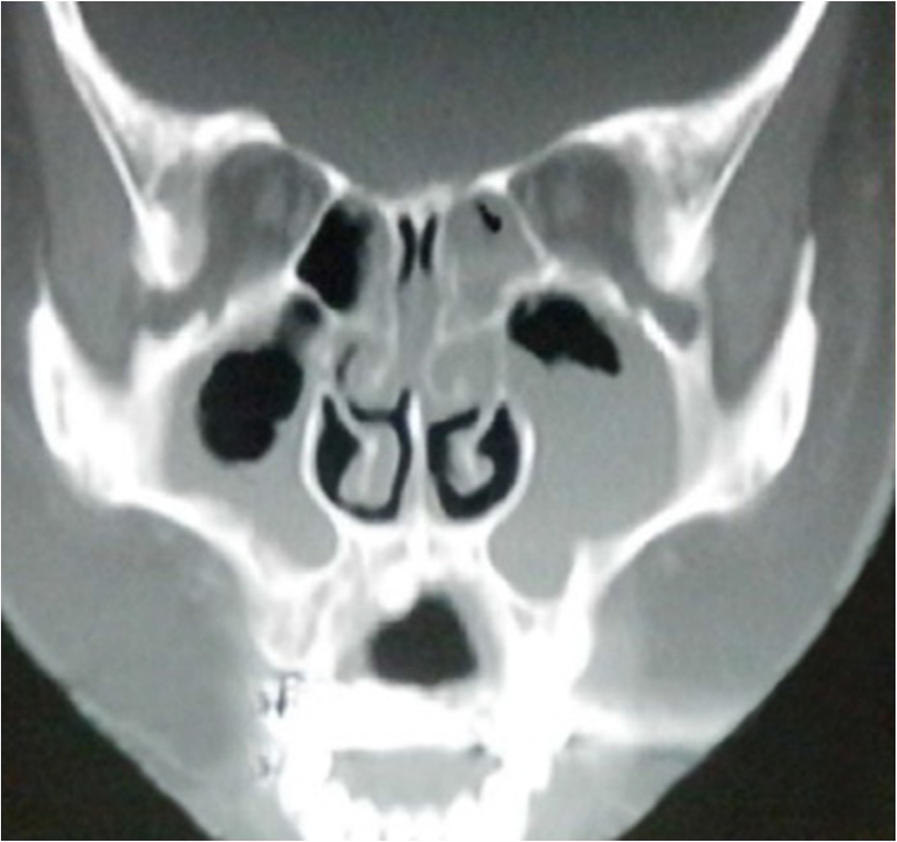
Sinuses computed tomographic scan of 39 years old woman after cardiac surgery. Sinuses imaging showed acute rhinosinusitis & homogenous parasinuses densities 20 days after miral valve replacement
